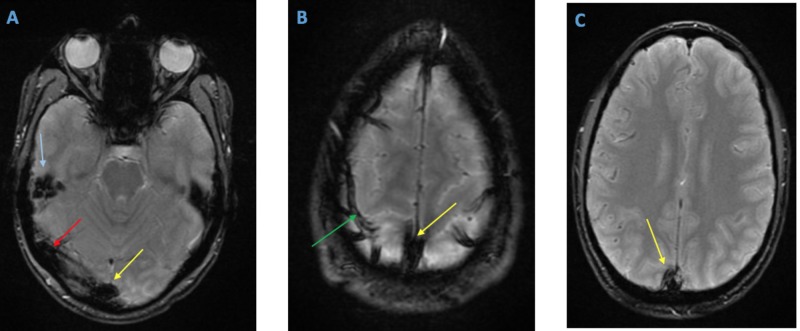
Magnetic resonance imaging (MRI) of the brain - axial gradient recall echo (GRE) sequence A, B, and C) MRI of the brain without contrast (GRE sequence, axial view) shows susceptibility changes demonstrating thrombosis of the superior sagittal sinus (yellow arrows), sigmoid sinus (blue arrow), right transverse sinus (red arrow), and cortical veins (green arrow).
